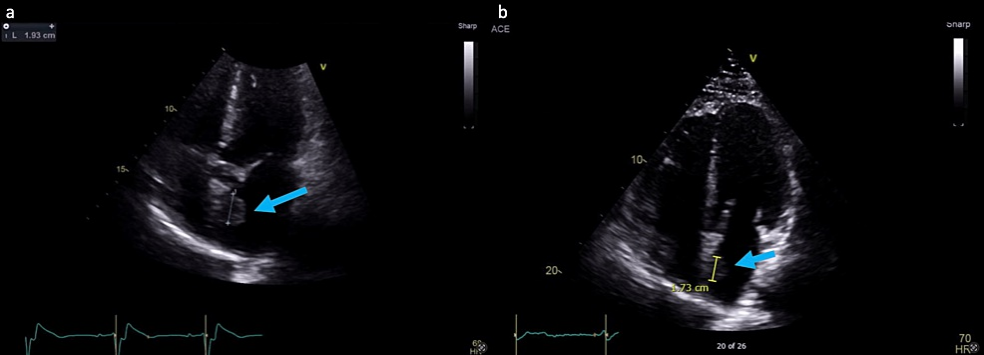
Transthoracic echocardiogram: (a) echocardiogram demonstrating a 1.9 cm left atrial mass and (b) echocardiogram performed three months prior demonstrating the left atrial mass measuring 1.7 cm (arrows).
